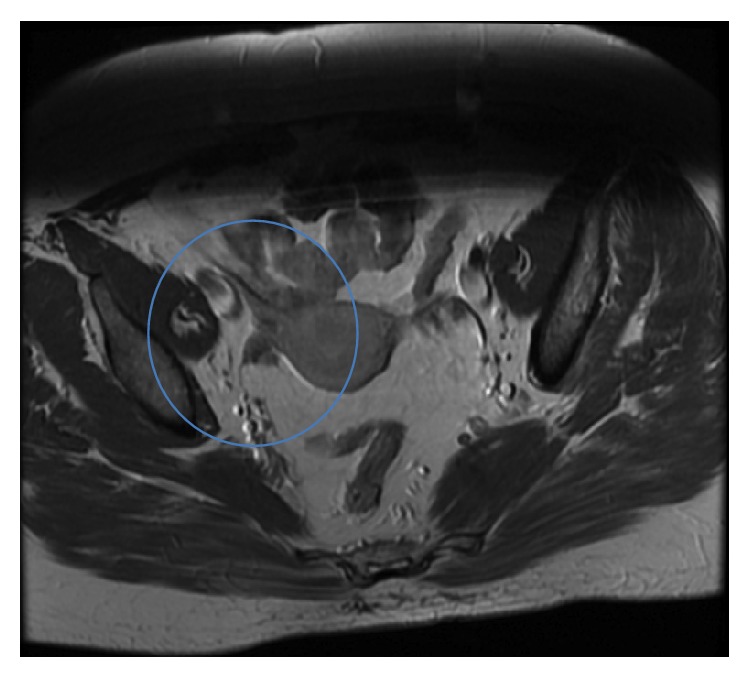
Follow-up MRI of pelvis at 4 years postpartum confirms sustained regression with no signs of tumour recurrence.
